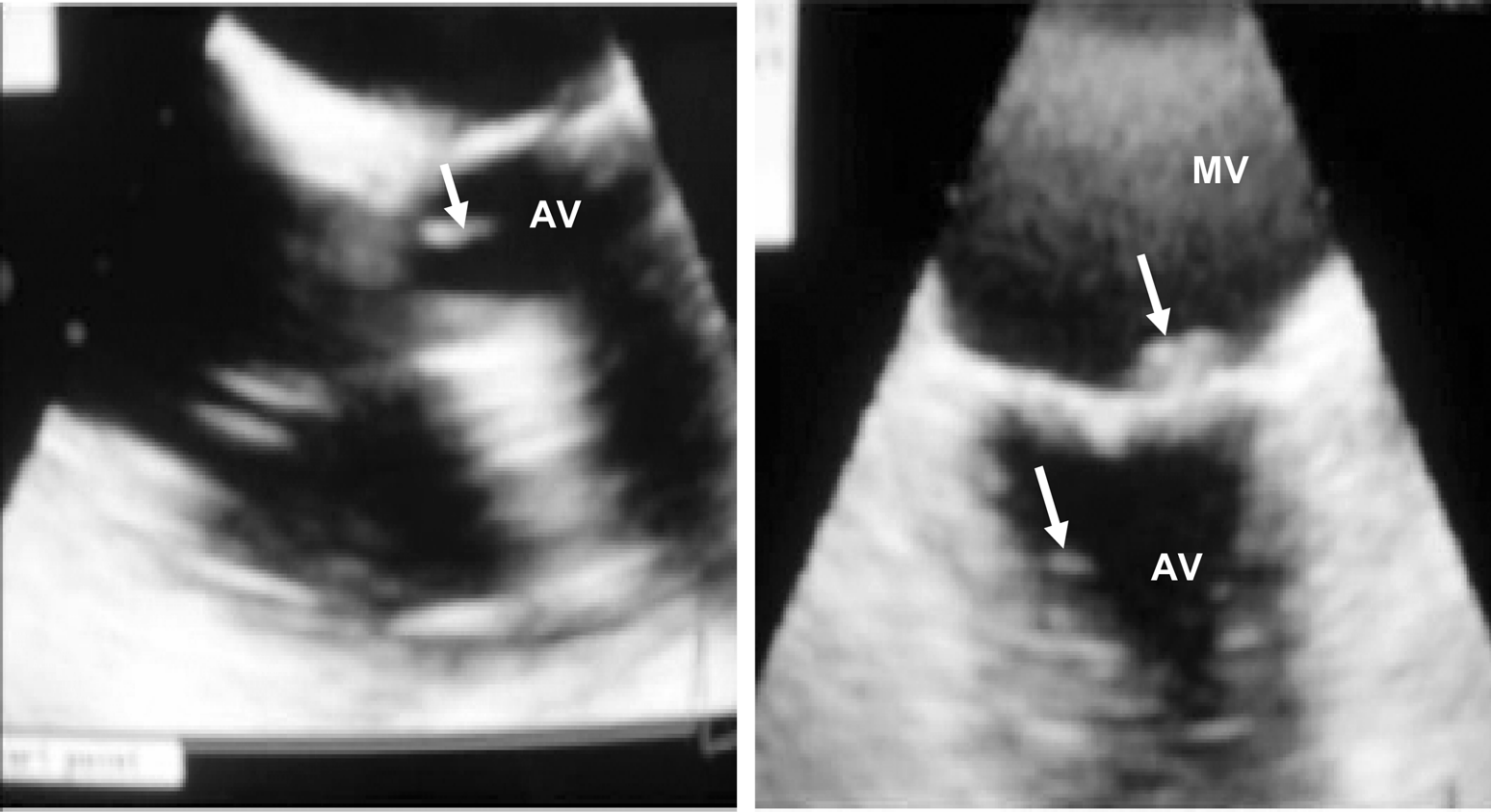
Transesophageal echocardiography of a patient with infective endocarditis caused by CA-MRSA with phenotype h-VISA, Argentina. Left: On April 12, the highly mobile vegetation on the aortic valve (AV) of 20 × 41 mm (white arrow). Right: On April 24, slight decrease in the aortic valve (AV) vegetation (white arrow) and a new vegetation on the mitral valve (MV) (10 × 4.6 mm) of low motility (white arrow).
